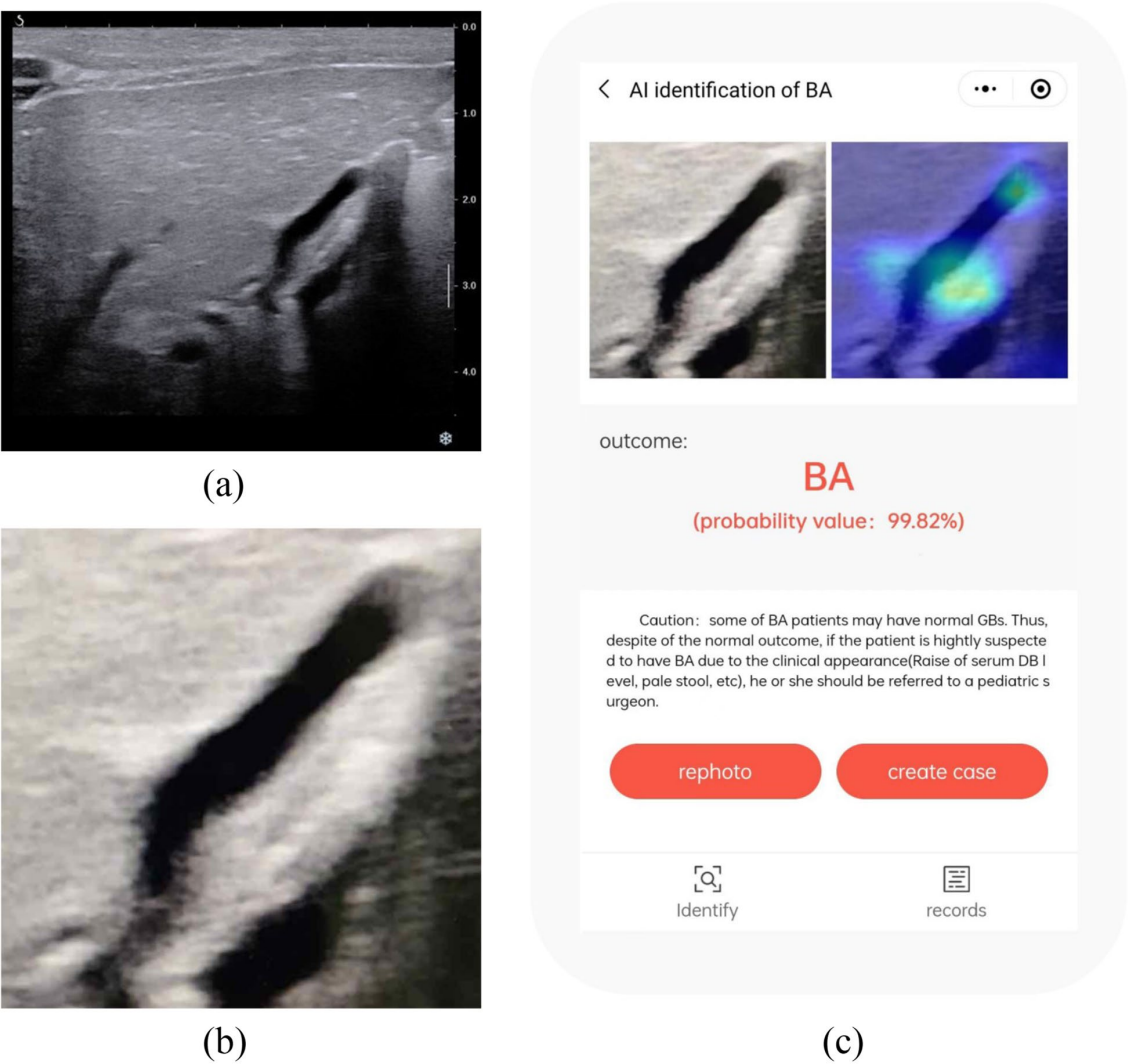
Example of a case where radiologists obtained the correct diagnosis with the assistance of the new smartphone app. a An original sonographic gallbladder image selected from the video of a 65-day-old female infant with biliary atresia. Seven radiologists considered the gallbladder to be normal. However, the diagnosis provided by the model obtained from the photos taken by six of these seven radiologists indicated biliary atresia, and the heatmaps all focused on the gallbladder. Finally, five radiologists revised the diagnosis of this infant to biliary atresia. b The smartphone photo taken by radiologist J. c The output interface of the test result in the new smartphone app for smartphone photo shown in b, displaying a probability value of 99.82%. With the assistance of the new smartphone app, radiologist J finally revised the diagnosis of this infant to biliary atresia
